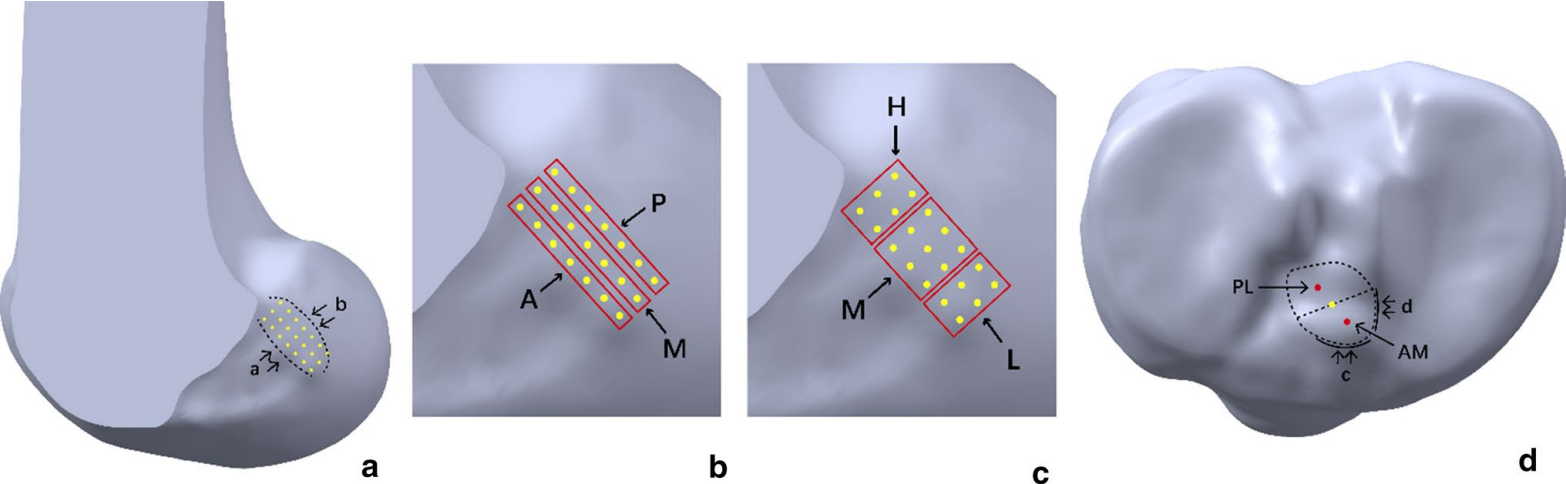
a The twenty-one femoral attachment points of the ACL on the femoral footprint. *a* Lateral intercondylar ridge; *b* the edge of femoral articular cartilage. b, c The different locations. *A* anterior location; *M* middle location; *P* posterior location. *H* high location; *M* middle location; *L* low location. d The tibial attachment point of the ACL that was identified based on the bony landmarks on the 3D CT models. *PL* posterolateral; *AM* anteromedial; *c* anterior ridge; *d* medial intercondylar ridge
